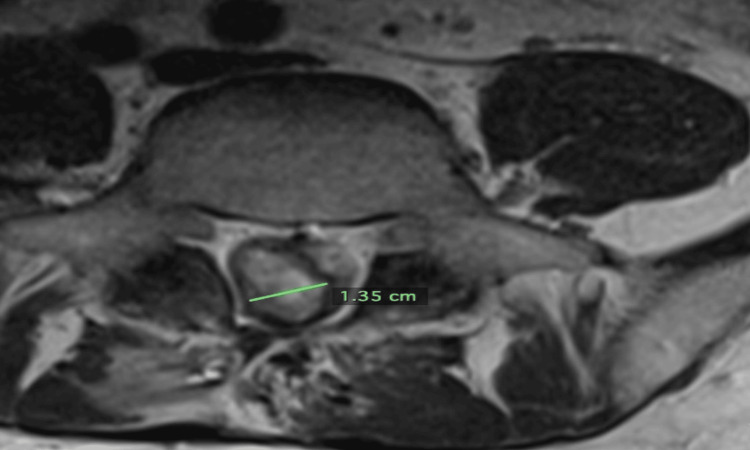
Pre-cryoablation axial image from an MRI L-spine dated May 3, 2023. The tumor measured 1.35 cm transversely.
